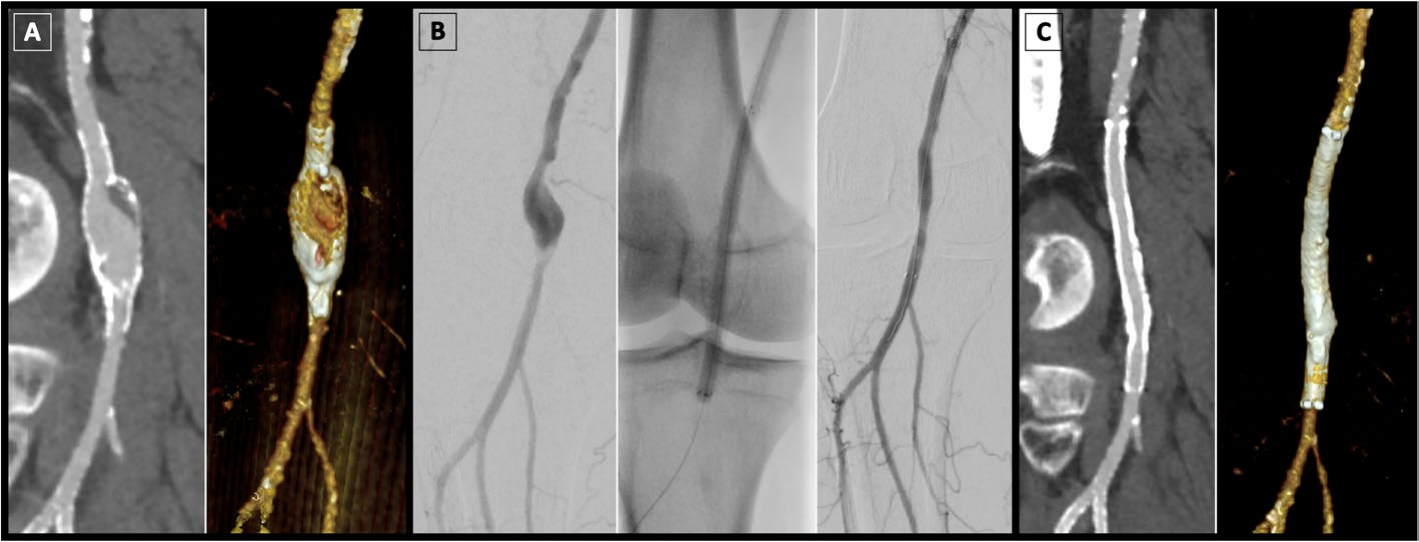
Viabahn endoprosthesis for endovascular treatment of popliteal aneurysm. Example of a 61-year-old man with peripheral artery disease and moderate claudication. A CT angiography (CTA) revealed a popliteal aneurysm and stent fracture in the P2 segment. B Subsequently, the patient underwent Viabahn stent graft procedure. Final target site angiography demonstrated successful aneurysm exclusion by the implanted Viabahn with preserved blood flow and no peripheral complications. C Follow-up CTA at 2 years demonstrated sustained blood flow through the device
